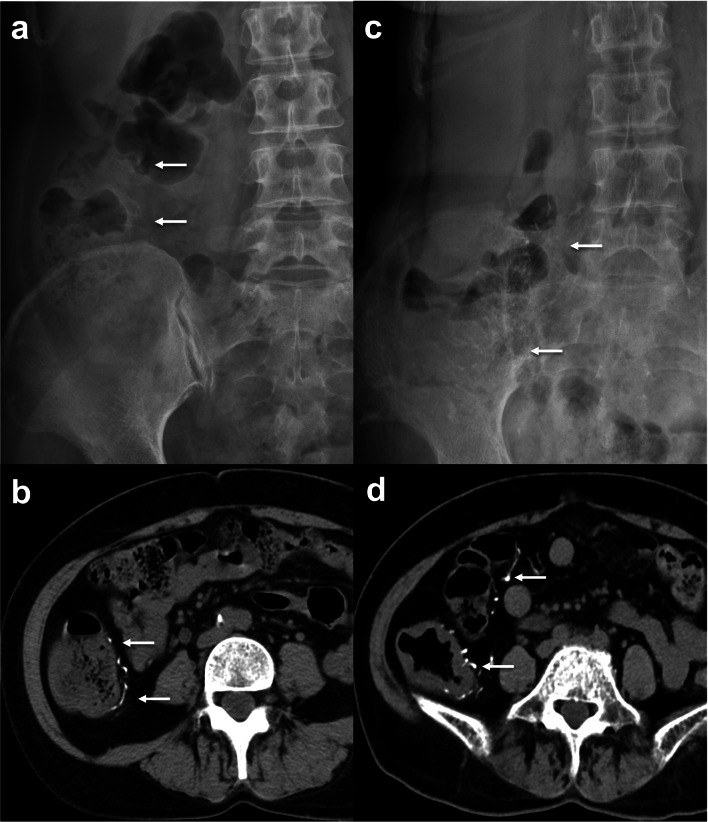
A 51-year-old woman with bladder cancer incidentally found mesenteric venous calcifications. a, b Plain radiograph and abdominal CT show curvilinear calcifications (arrows) of the straight veins of the cecum and proximal ascending colon. c, d Intermittent diarrhea noted for 7 years and follow-up radiograph and CT show progression of mesenteric venous calcifications (arrows) and cecal wall thickening without pericolic inflammation
